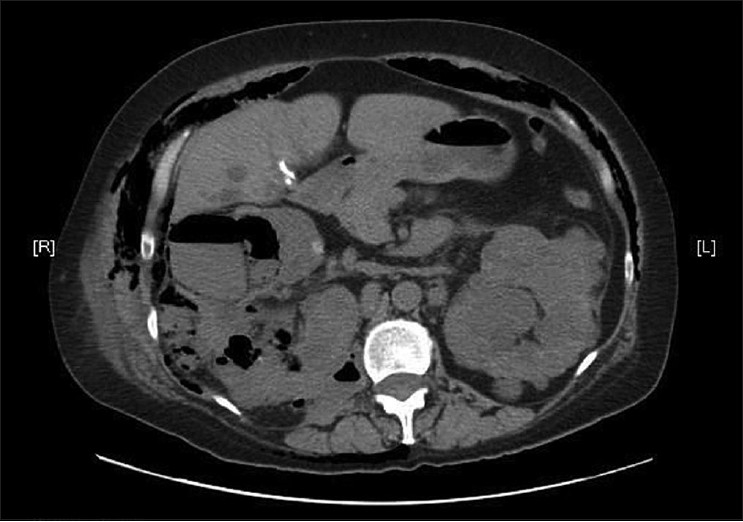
EPN on CT of abdomen; the subcutaneous emphysema extending from the abdomen's right side involves the thorax
